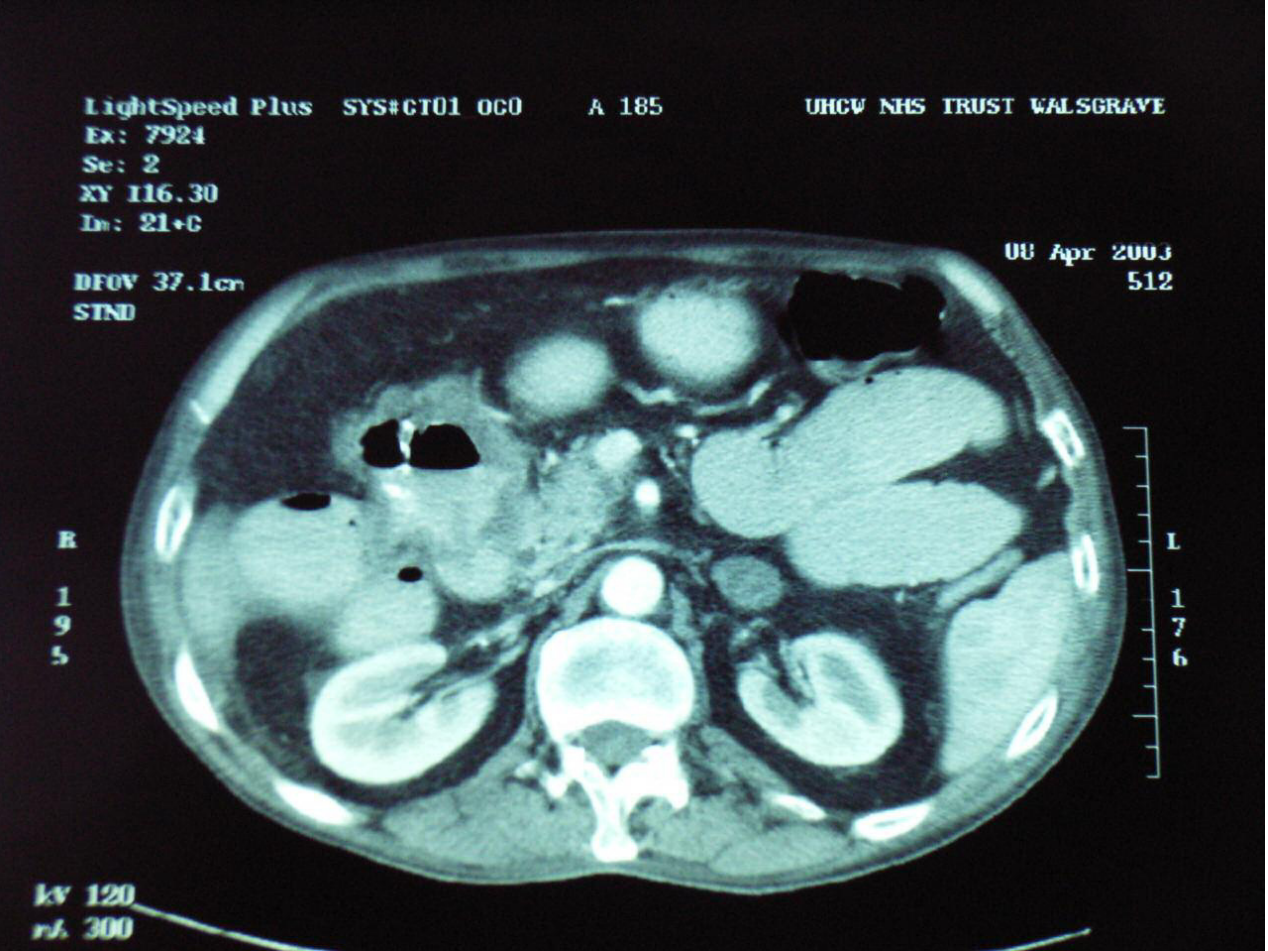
Abdominal CT showing a locally advanced tumour in proximal transverse colon with a fistula into the second part of duodenum.
